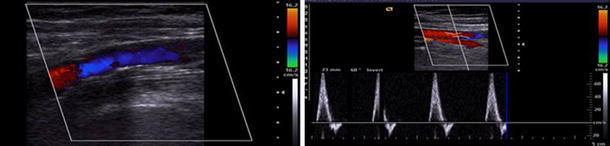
Doppler sonography. Severe luminal stenosis associated with diffuse atherosclerotic and calcified plaque
